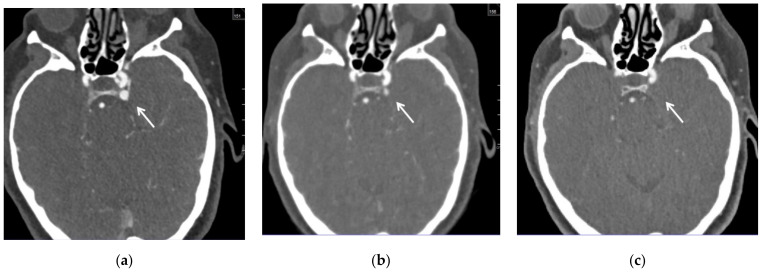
CT angio on admission (a) and at 3 (b) and 6 (c) month follow-up. The venous pouch at the posterior aspect of the left cavernous sinus (white arrow) progressively decreased in size and disappeared.
